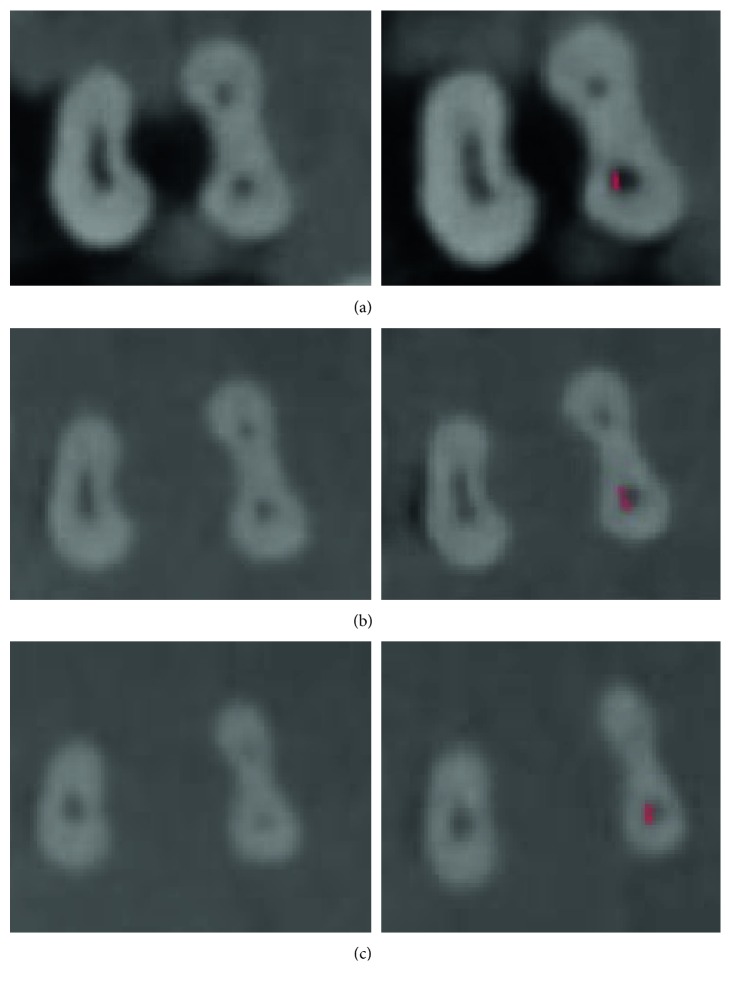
CT scan images before (left column) and after (right column) instrumentation with One Shape. Red marks indicate canal transportation. (a) Coronal third, (b) middle third, and (c) apical third.
